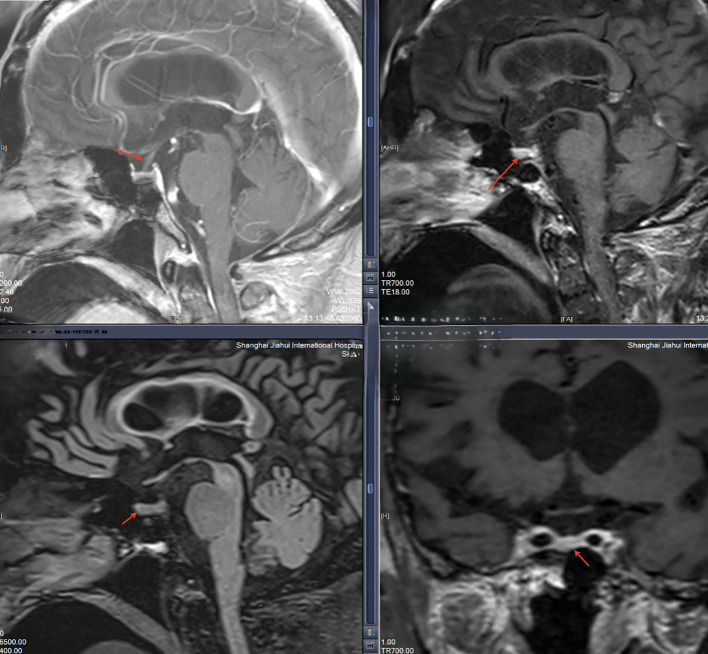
January 12, 2026 Brain MRI showed slight pituitary stalk thickening.
